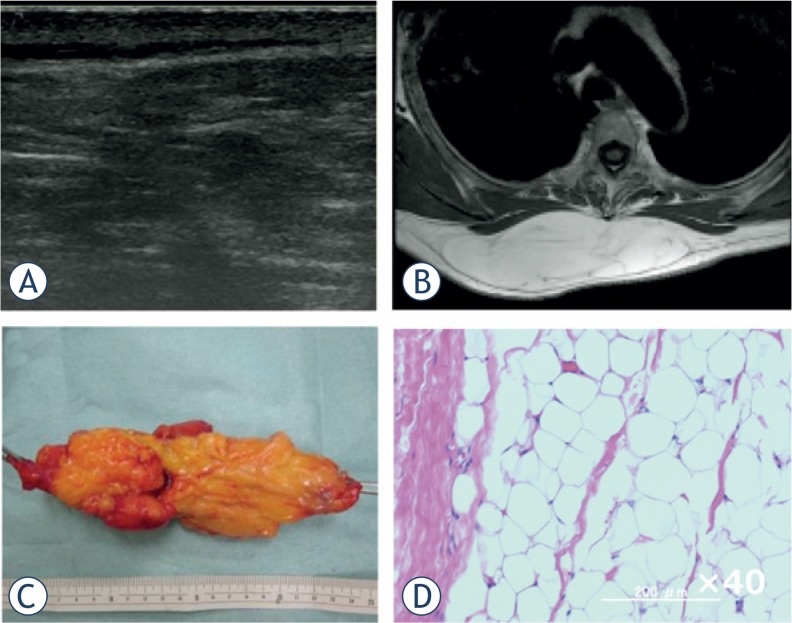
Subcutaneous lipoma of the back. A 55-year-old woman presented with a subcutaneous mass on her back. (A) Ultrasound revealed a highly echoic mass with a mixed inner texture and no intratumoural vessels by Doppler echo. (B) MRI showed a high-intensity mass within the subcutaneous fat tissue on T1-weighted images. (C) Gross appearance of the marginally resected tumour. (D) Pathological examination of the tumour revealed mature adipocytes with no atypical cells.
